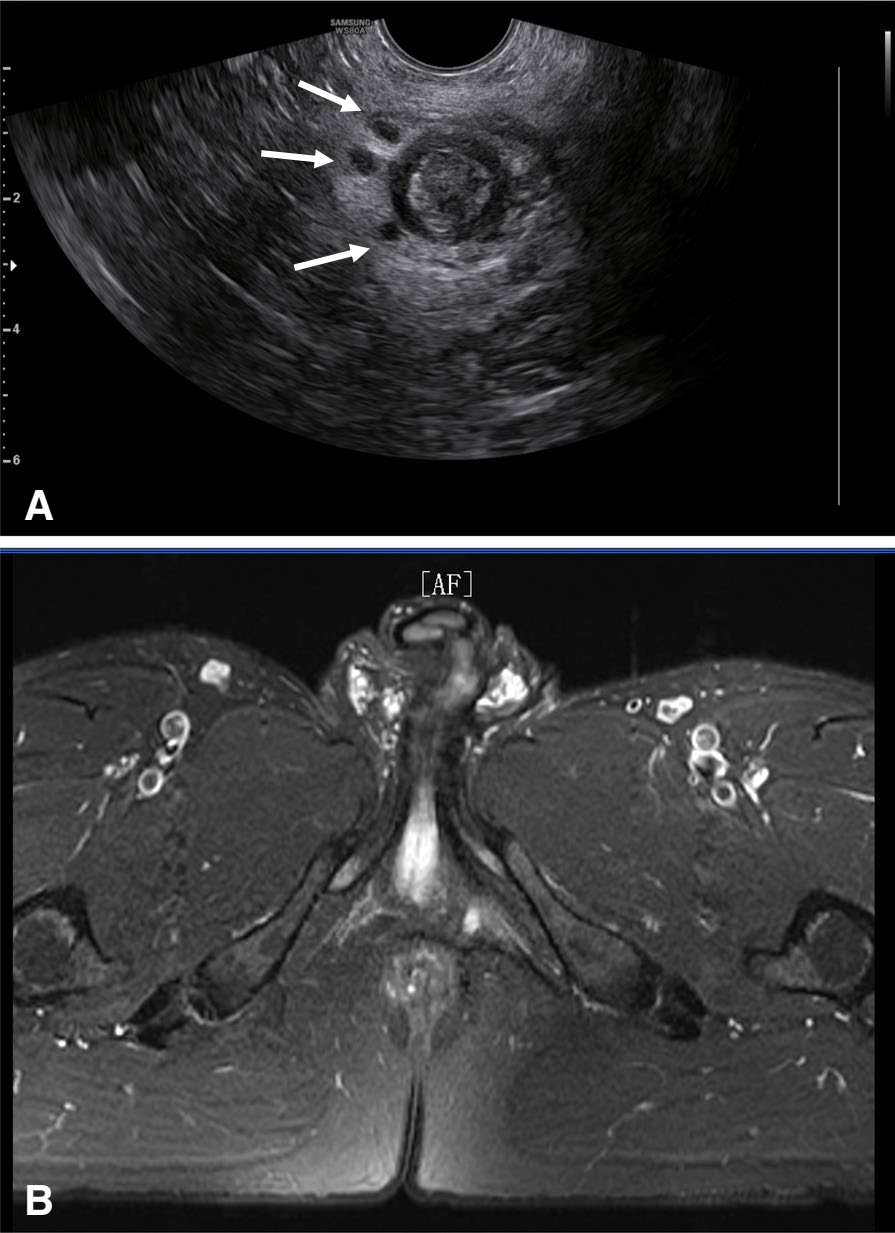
Anal fistula type II and IV in pelvic 3D US and MRI images. A Pelvic 3D US image of internal openings(white arrow); (B)MRI image of a anal fistula
